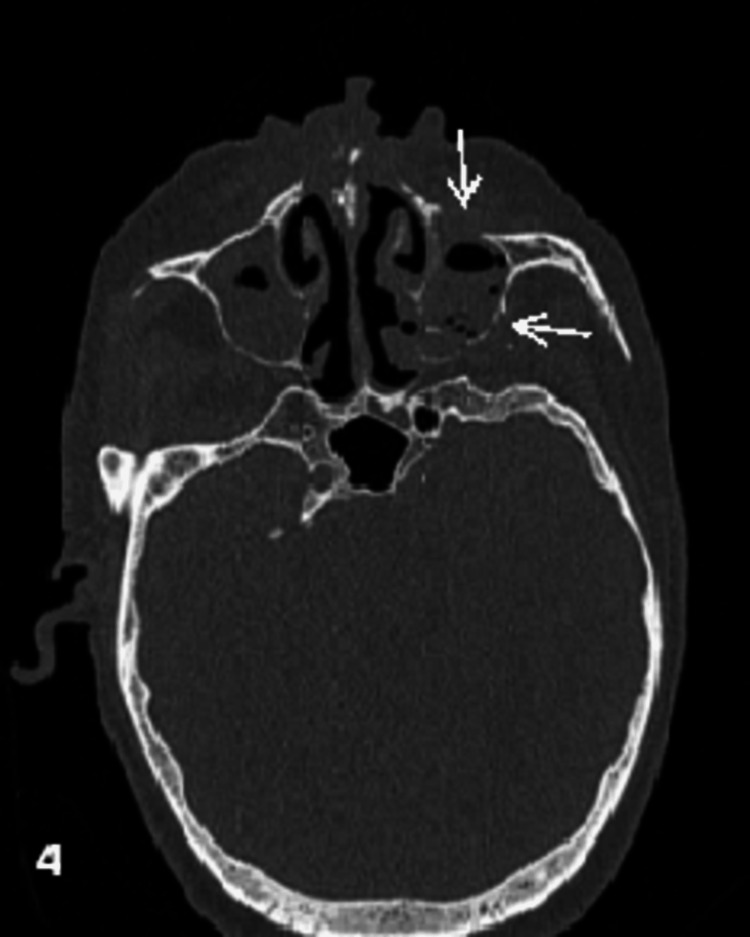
Osseous involvement Axial CT bone window image of a 65-year-old diabetic patient who presented with facial pain and redness showing erosion of the anterior and posterior wall of the left maxillary sinus (arrows). CT: computed tomography
